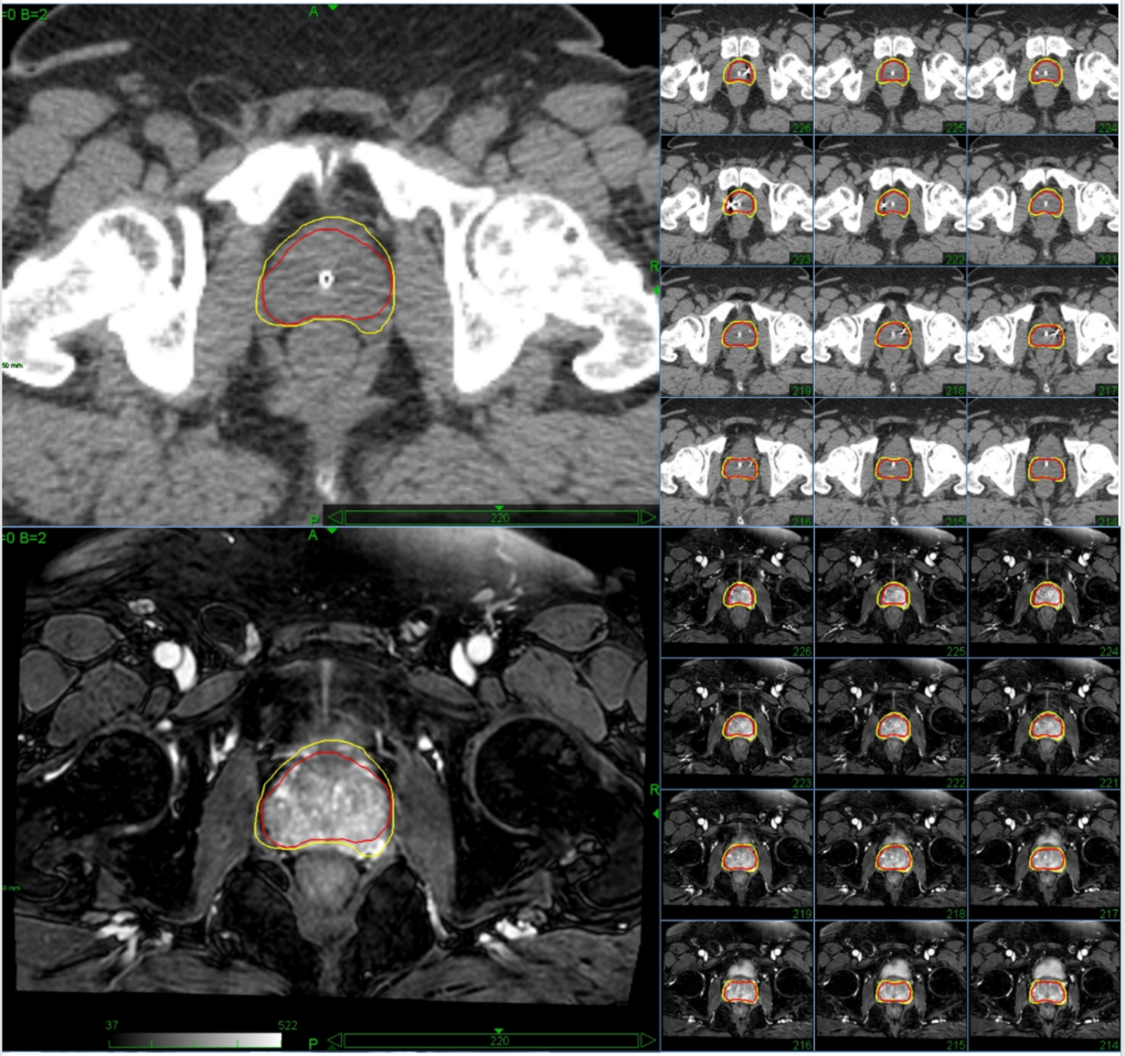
Comparing the contours of the prostate using CT (top) vs. MRI (bottom) Yellow = Contour of the prostate using computed tomography (CT) Red = Contour of the prostate using open magnetic resonance imaging (MRI)
